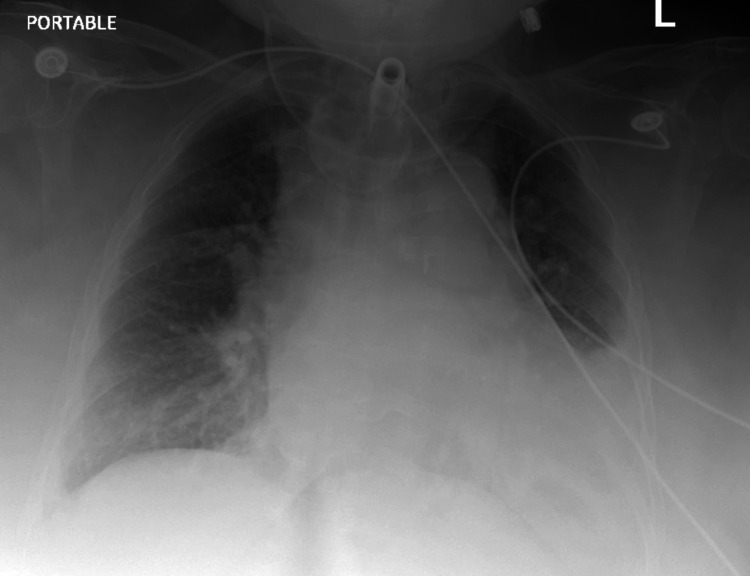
Chest X-ray at the time of admission revealing bilateral perihilar markings suggestive of pulmonary consolidation and/or edema Blunting of left mid and lower lung fields as well as the right costophrenic sulcus indicating pleural fluid.
